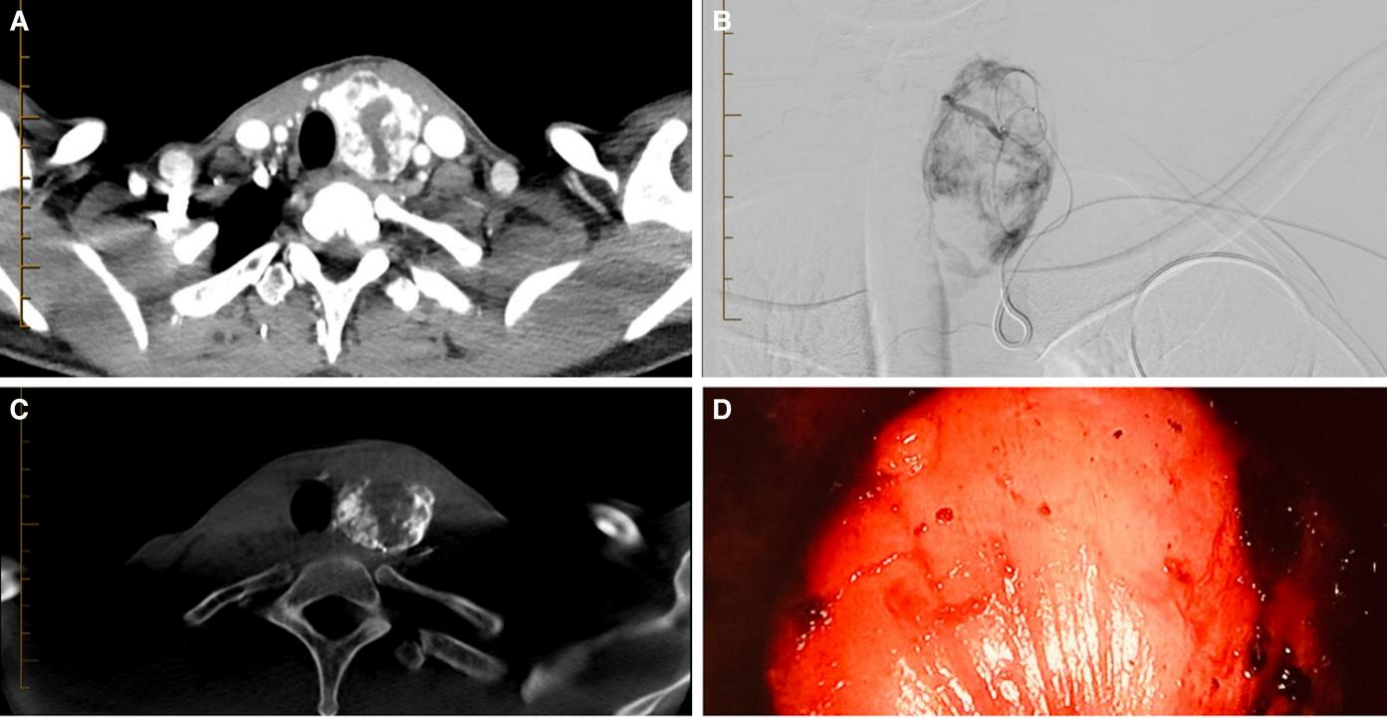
Presurgical embolization case. A 29-year-old woman presenting with a Bethesda 3 nodule measuring 20 mL in volume and associated PAX8 and PPARG mutation (70% risk of malignancy) and offered transoral thyroidectomy. A, Axial computed tomography (CT) of the neck with contrast demonstrates a hypervascular left lobe nodule. B, Angiography following catheterization of the left inferior thyroid artery with the pressure-enabled drug delivery device with the device positioned after the first turn of the artery demonstrates a significant hypervascular nodule without arterial reflux. C, Axial image obtained during intraprocedural cone beam CT acquired in the angiography table, demonstrates a peripherally hypervascular soft tissue lesion within the left lobe of the thyroid corresponding with the findings of the preprocedural CT. D, Direct endoscopic visualization of the left thyroid lobe. Note the pale appearance and the overall devascularization of the left lobe of the thyroid following embolization prior to resection.
